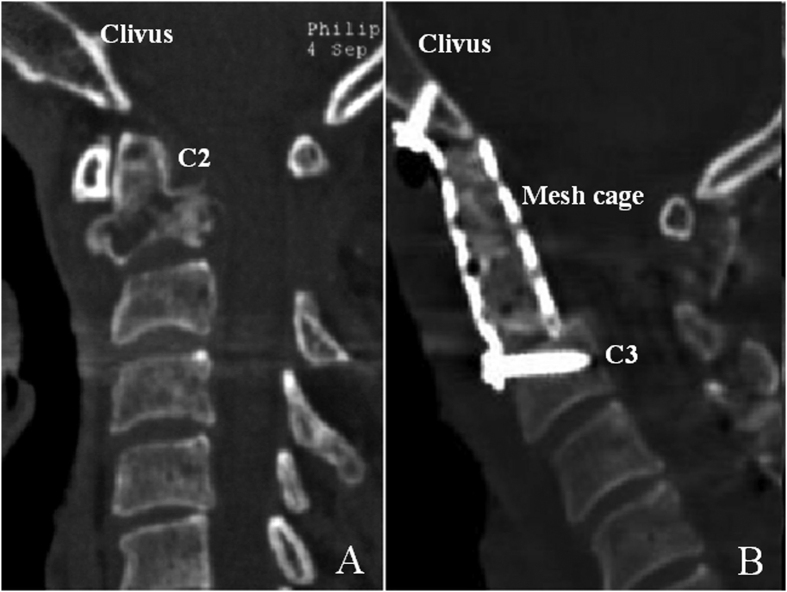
Preoperative sagittal (A) reconstructed CT images of the cervical spine with an osteolytic destruction of C-2; Underwent a single-stage total C-2 intralesional spondylectomy, a postoperative sagittal (B) reconstructed CT images of the cervical spine showing the mesh cage screwed into the clivus cranially and C3 vertebral body caudally^13^. C2, the second cervical vertebra; C3, the third cervical vertebra.
